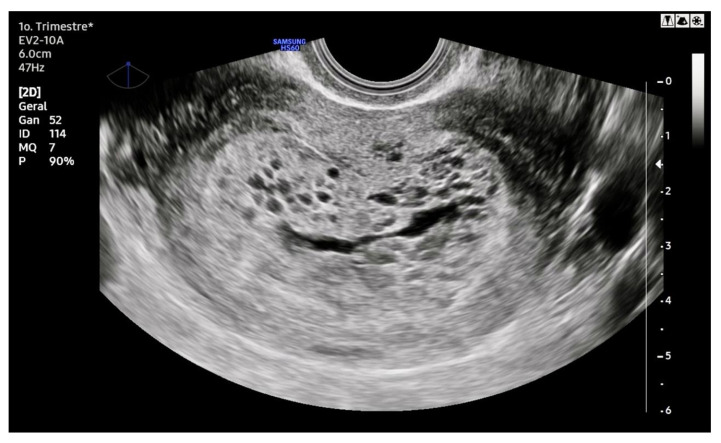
Ultrasound of complete hydatidiform mole. The endometrial cavity is filled with an anechoic mass exhibiting multiple cystic areas (representing hydropic vesicles). No embryonic or appendageal structures are seen, but the uterus appears enlarged for gestational age, measuring 12 cm at its greatest diameter in what corresponds to an 8-week pregnancy.
